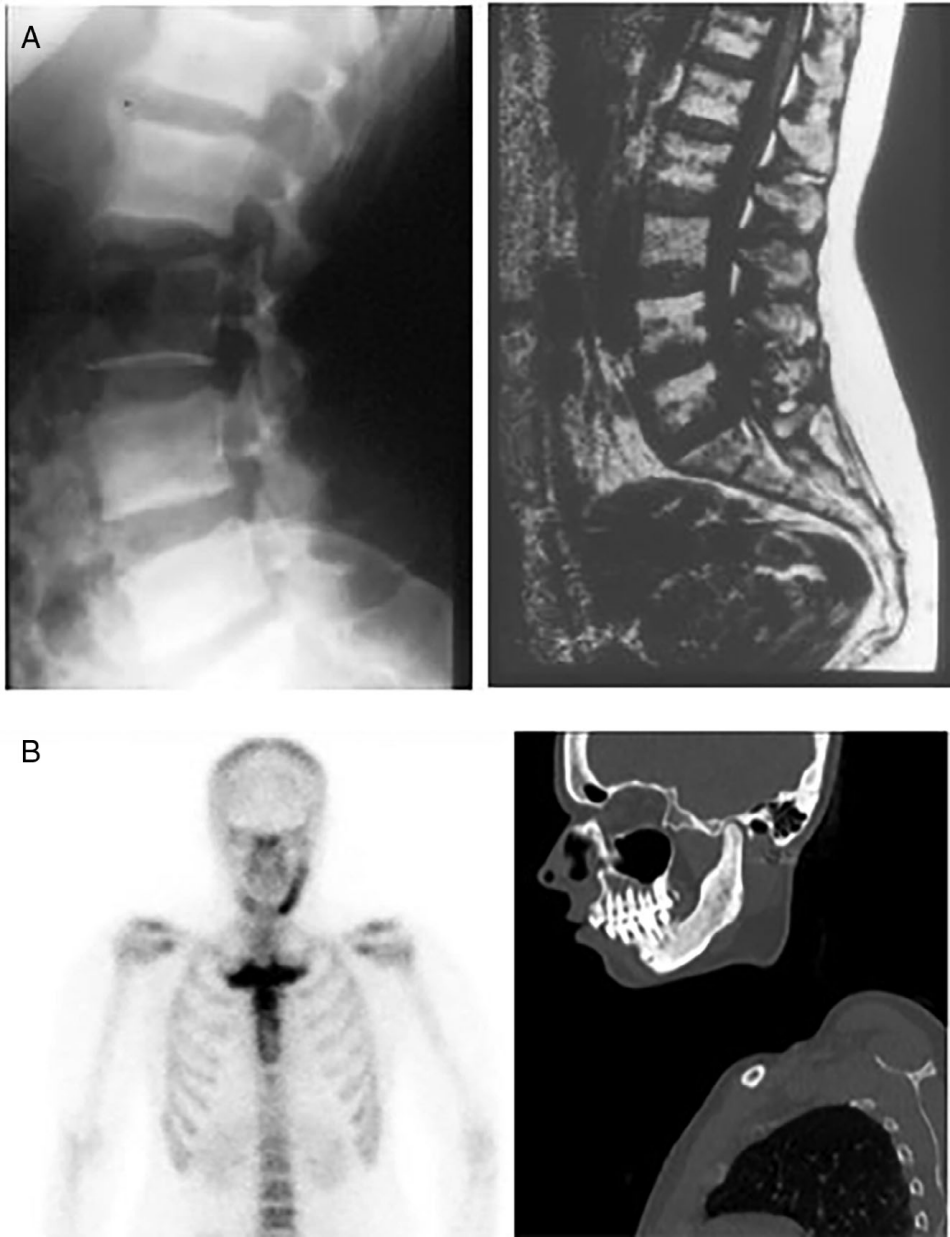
(*A*) Other axial localizations in chronic nonbacterial osteomyelitis/sternocostoclavicular hyperostosis (CNO/SCCH); multiple level vertebral involvement in a patient with CNO/SCCH showing irregular sclerosis of multiple vertebrae especially at the end plates and starting ossification of anterior ligaments, with only the third lumbar vertebra being spared on plain lateral radiographs of the spine on the left, as also confirmed on MRI on the right. (*B*) Mandibular localization of chronic nonbacterial osteomyelitis.
